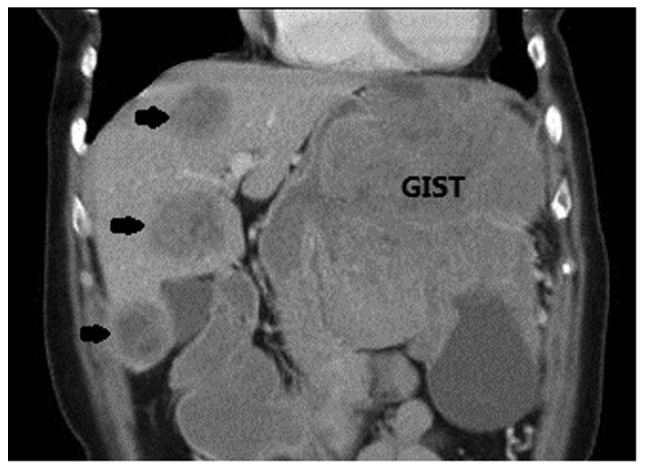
Enhanced CT scan (coronal reconstruction) showed a giant mass in the stomach and three low-density regions in different liver segments (arrow) with evident heterogeneous enhancement. CT, computed tomography; GIST, gastrointestinal stromal tumor.
